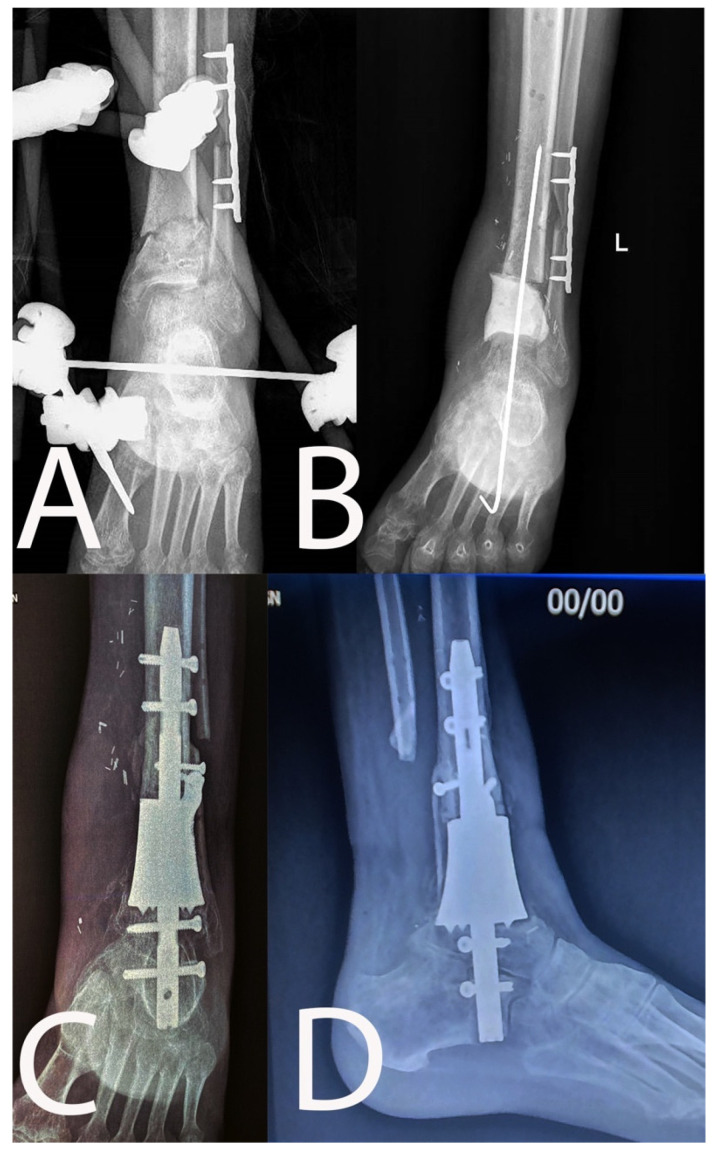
Chronic osteomyelitis of the distal tibia with non-healed pseudoarthrosis ((A): pre-operative anteroposterior X-rays) treated with resection and antibiotic-loaded spacer ((B): anteroposterior X-rays). Reconstruction was performed in ankle arthrodesis using a silver-coated custom-made prosthesis (7 months follow-up X-rays, (C): anteroposterior view, (D): lateral view).
